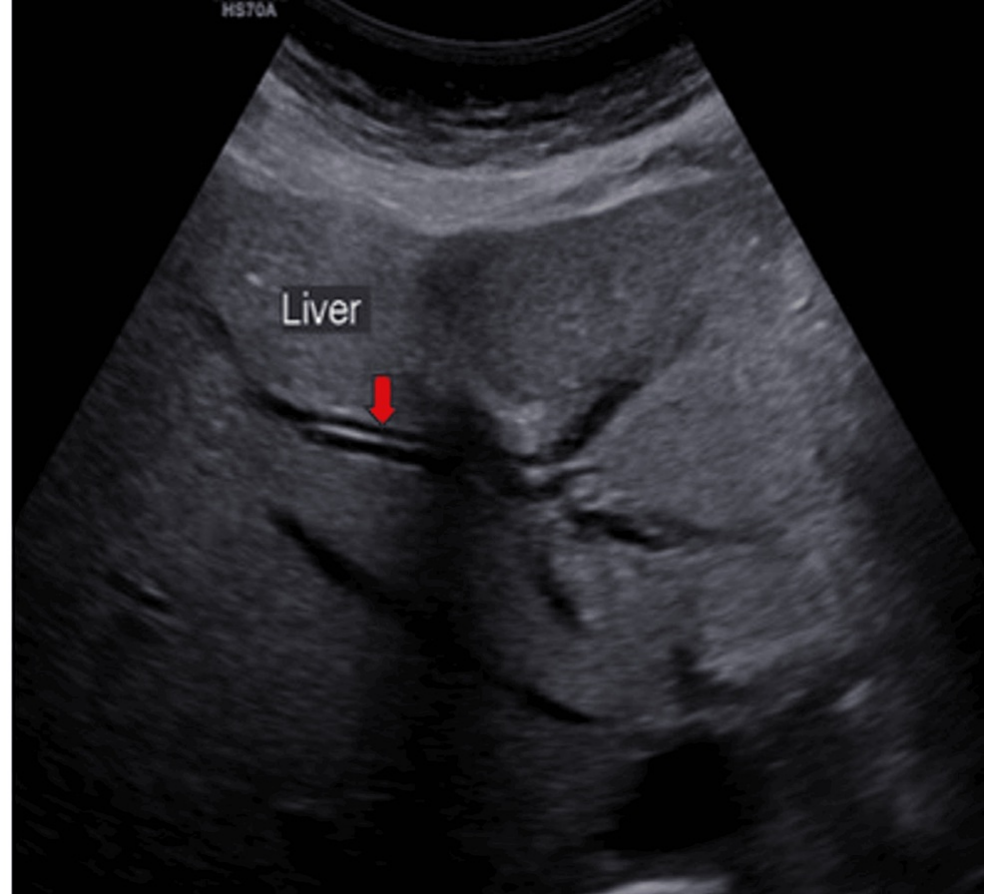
Ultrasonography image of the liver showing IHBR dilatation (red arrow). IHBR: Intrahepatic biliary radicle
